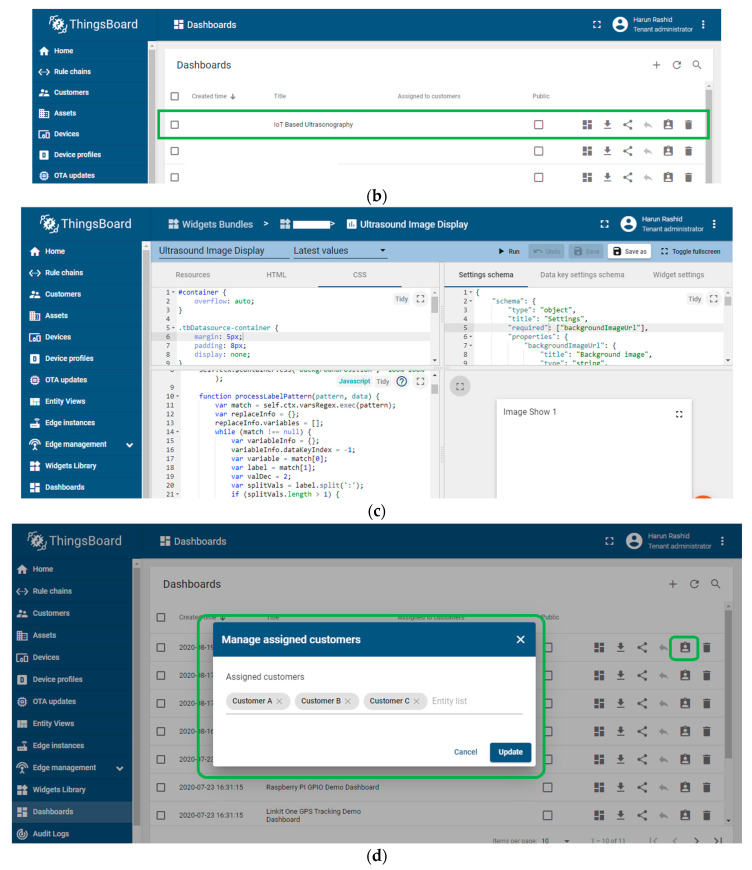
Thingsboard configuration: (a) Thingsboard dashboard (Main); (b) ultrasound machine dashboard. (c) Displaying images using a custom widget; (d) assigning user-based dashboards (access control).
